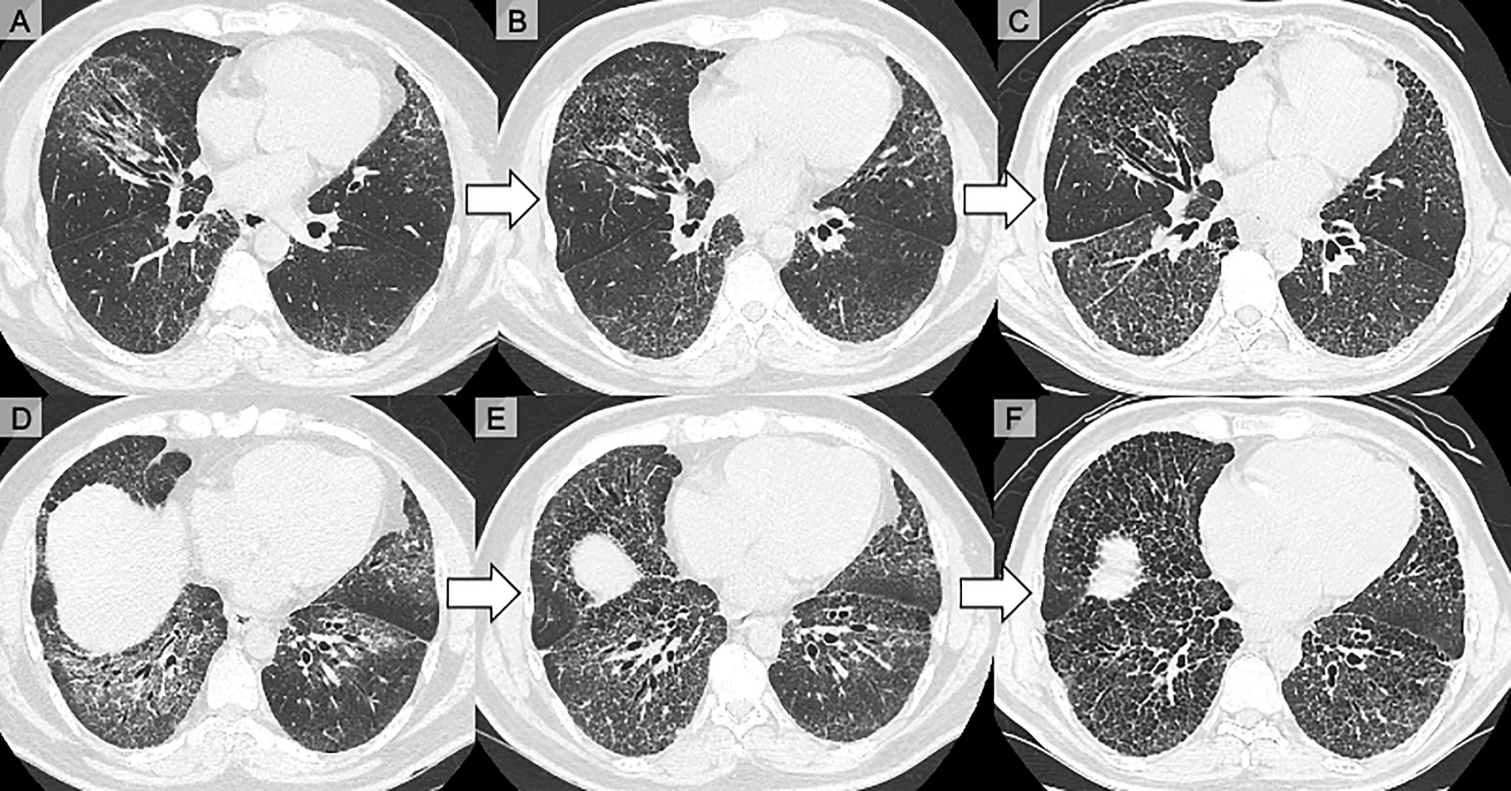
Serial chest computed tomography at two axial levels across three time points (days 1, 218 and 582). (A–C) Same axial slice on days 1 (A), 218 (B) and 582 (C). Bilateral basilar, predominantly peribronchial reticulation with traction bronchiectasis and reduced lung volume was observed on day 1; there was interval progression of fibrotic change by day 218, and further progression with increased reticulation, traction bronchiectasis and honeycombing by day 582. (D–F) Second axial slice on days 1 (D), 218 (E) and 582 (F) demonstrates the same basal‐predominant pattern with stepwise progression.
